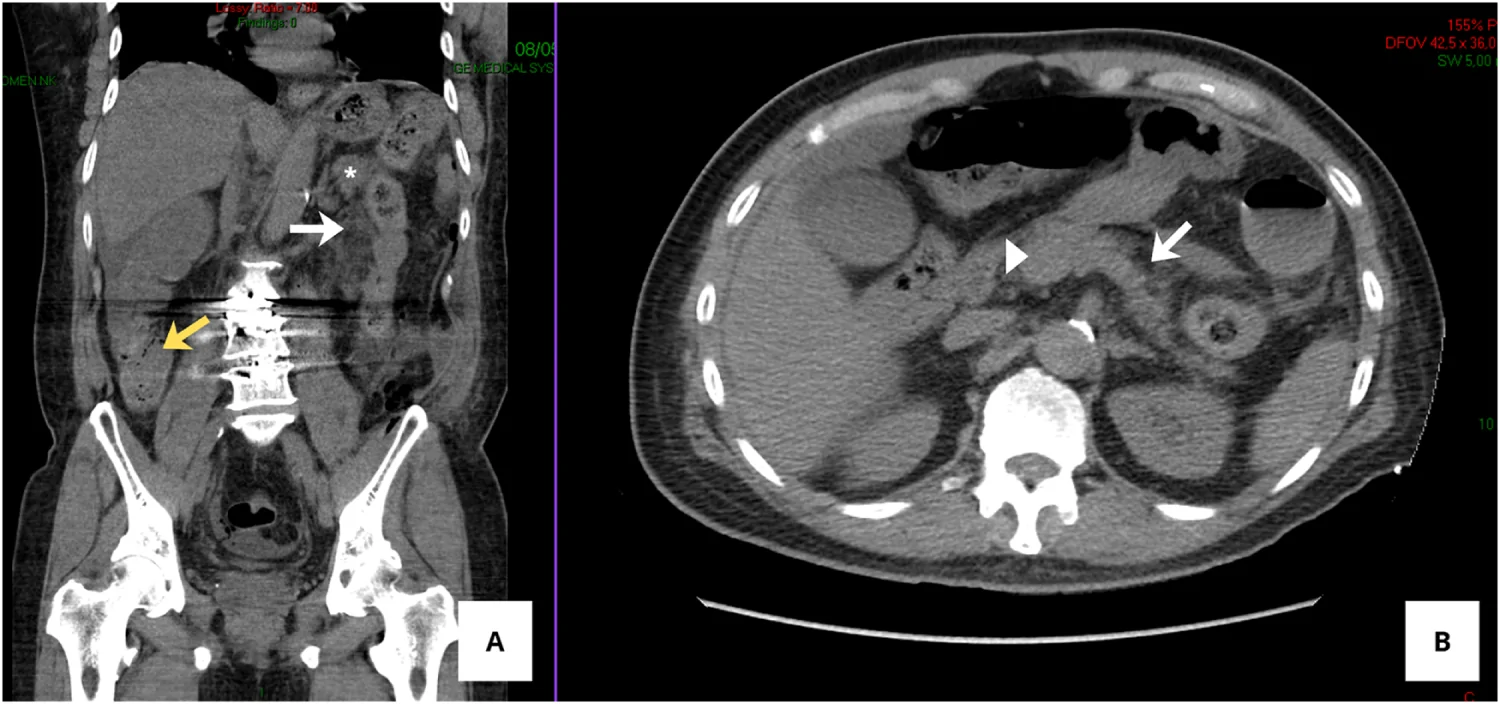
Non-contrast CT scan showing (A and B) an axial and coronal view of acute pancreatitis with pancreatic enlargement (white head arrow) and peripancreatic fat stranding (white arrow) around the body (white head arrow) and tail region (asterisk). There is also a small diverticulum at the ascending colon (yellow arrow) with no inflammatory changes.
